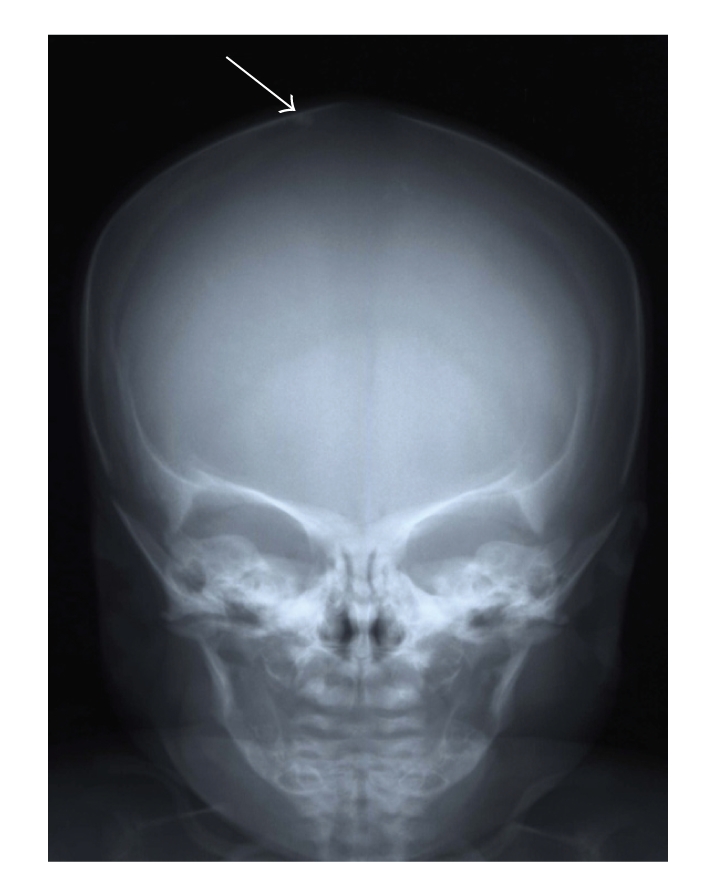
Skull radiograph of patient 1 taken on 13 days of age. White arrow indicates the calcification at right parietal region.
